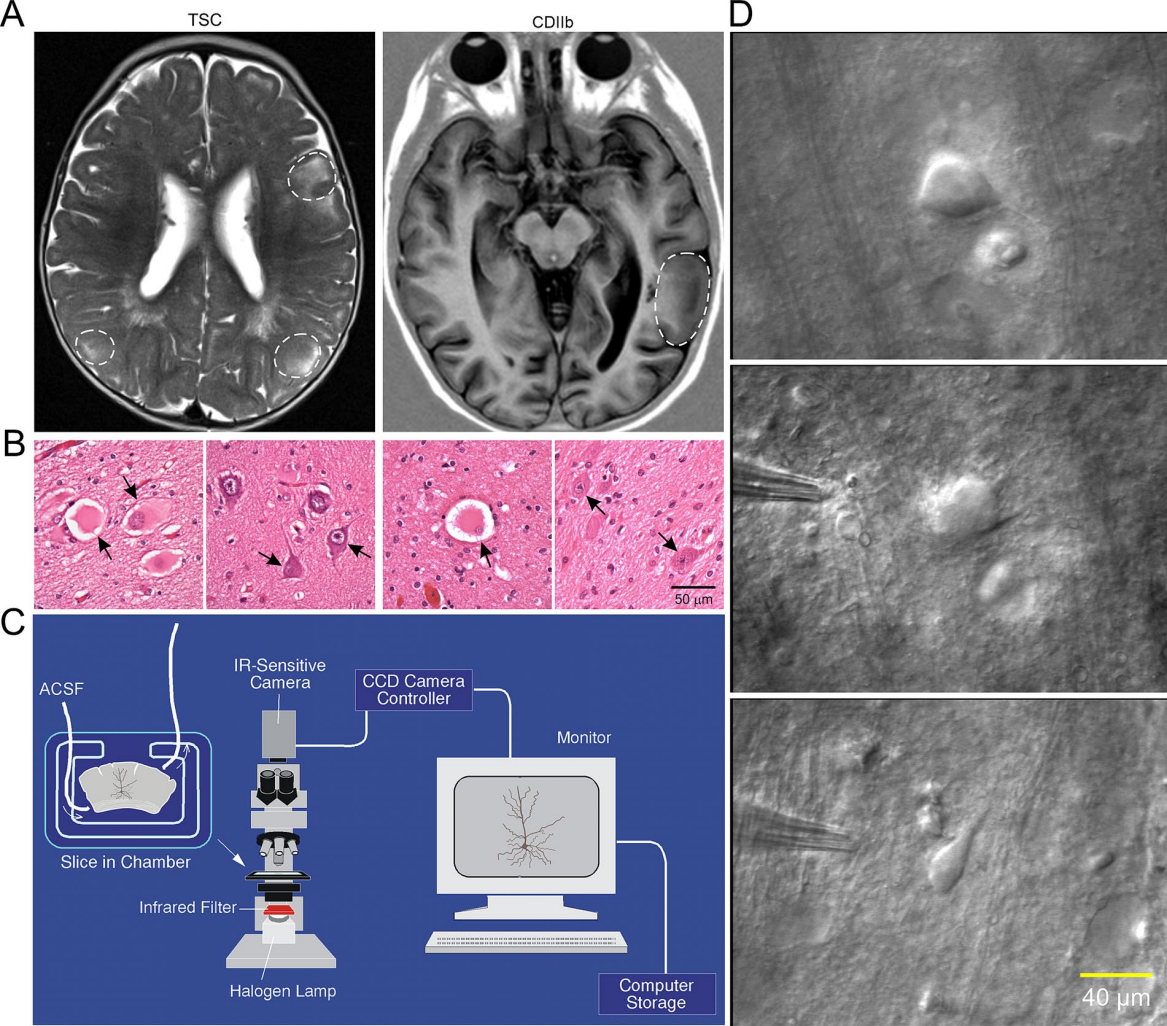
(A) Representative axial MRI scans of children (both 7 years old) with refractory epilepsy from TSC (left) and FCDIIb (right). There are multiple cortical tubers (dashed circles) in the patient with TSC and one focal area of cortical dysplasia in the left temporal lobe in the child with FCDIIb. (B)
